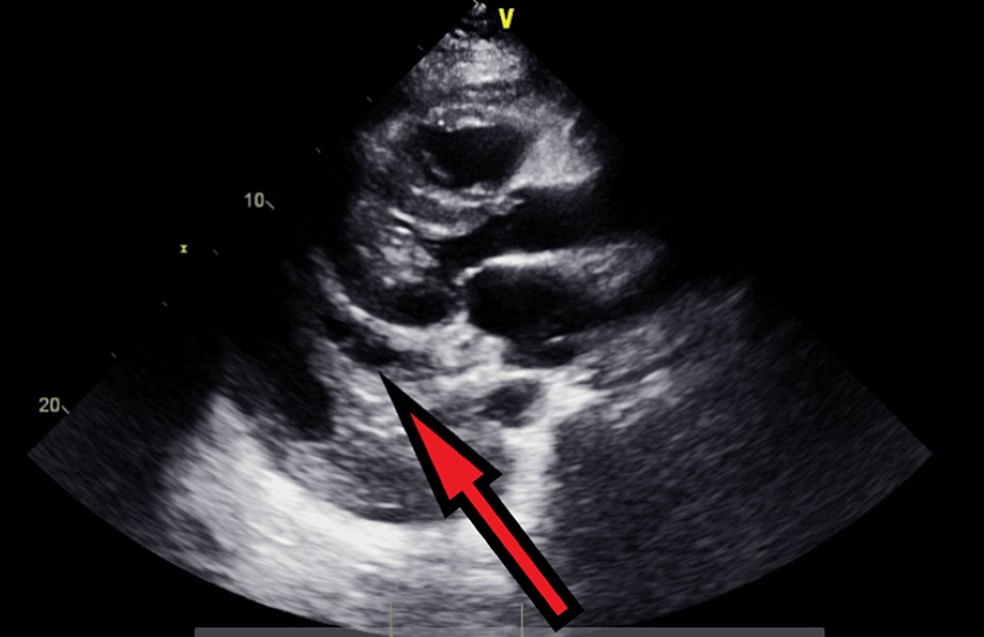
Transthoracic echocardiography post-pericardiocentesis - image 1 Transthoracic echocardiography post-pericardiocentesis; parasternal long axis view shows a decreased amount of pericardial fluid collection (red arrow)
